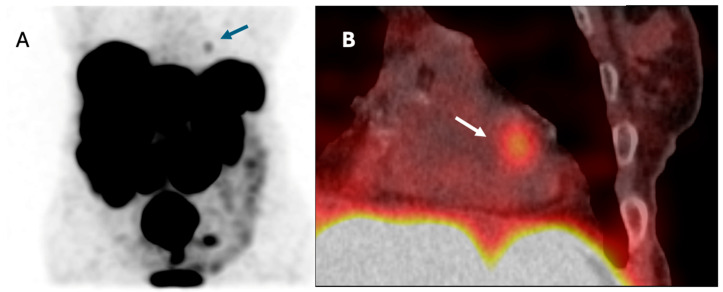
Post-therapeutic [^177^Lu]Lu-DOTA-TATE scintigraphy. (A)—[^177^Lu]Lu-DOTA-TATE planar scintigraphy, (B)—[^177^Lu]Lu-DOTA-TATE SPECT/CT. The cardiac metastasis is indicated by an arrow.
